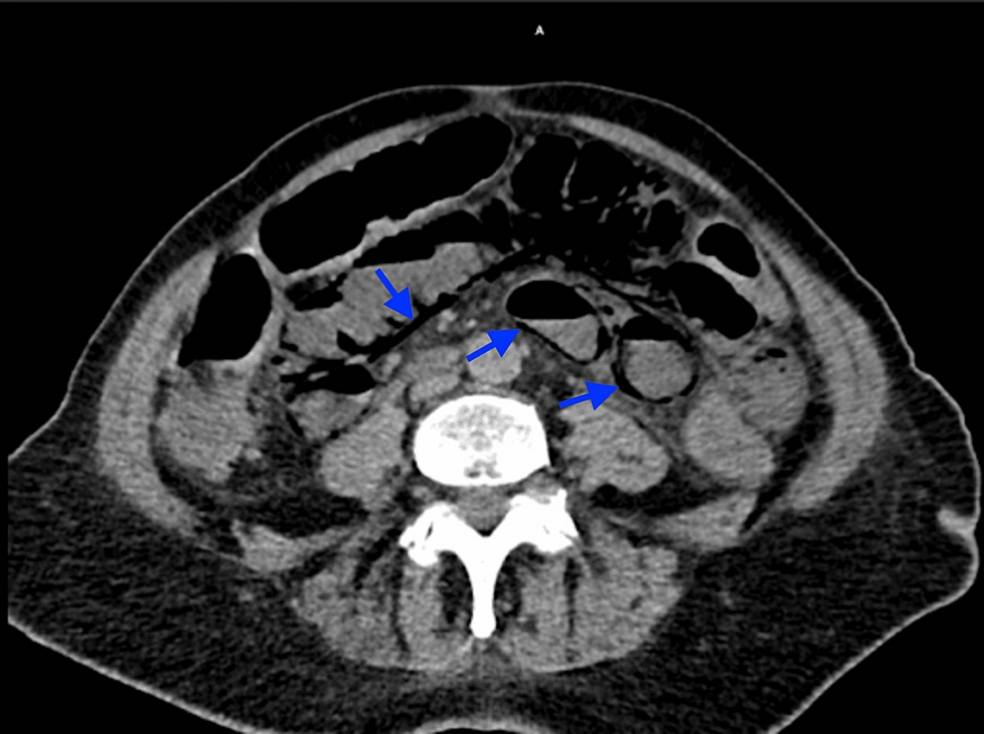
CT Abdomen/Pelvis without contrast (axial view) Pneumatosis intestinalis (shown by the blue arrows) within the proximal portion of the small bowel, the middle portion of the small bowel, and the transverse colon as a consequence of ischemic colitis.
